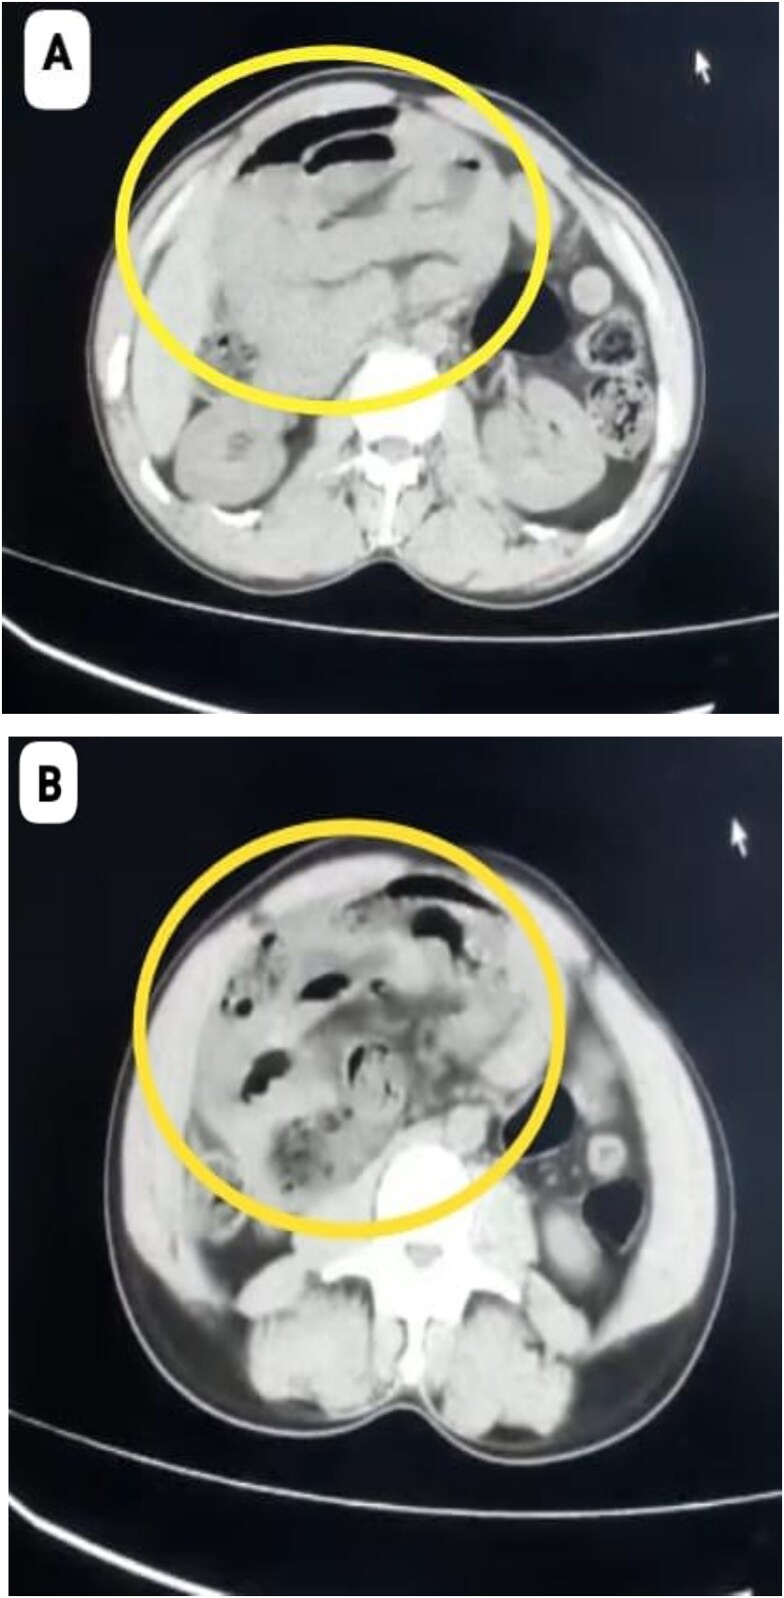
(A, B) CT Showing cluster of small bowel loops in right upper quadrant of abdomen consistent with right paraduodenal hernia.
